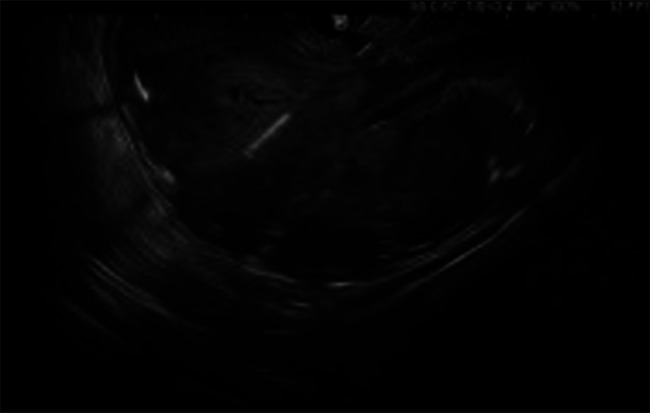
Endoscopic ultrasound showing hypoechoic and heterogeneous pedunculated mass in the cardia, fundus, and body of the stomach, measuring 100 mm by 100 mm in maximal cross-sectional diameter, with well-defined endosonographic borders.
